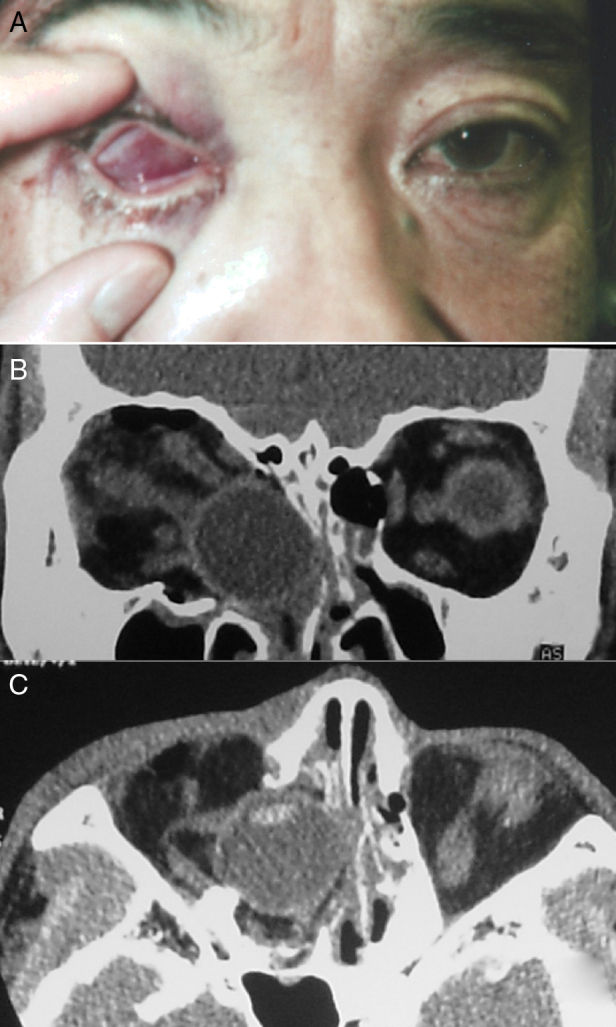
(A) Appearance of the right eye at initial presentation. (B) and (C) Fractures of the orbital floor and medial wall with a fallen globe were identified on computed tomography.
